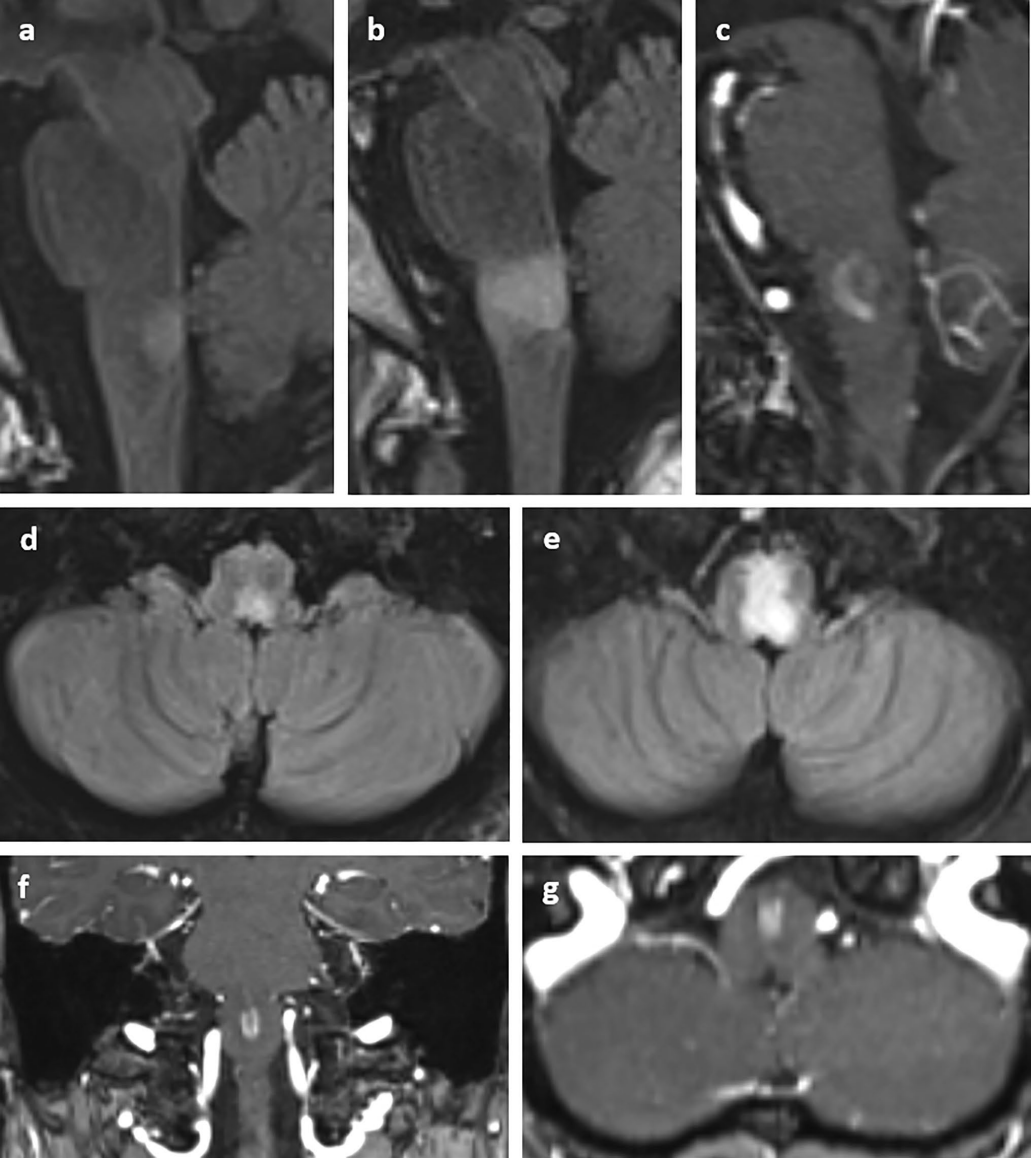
Cranial MRI 5 days after the onset of neurological symptoms revealed a T2/FLAIR-hyperintense lesion without gadolinium enhancement (not shown) in the dorsomedial medulla, localized in the nucleus tractus solitarii (a) Follow-up MRI 25 days later at the time of neurological deterioration showed enlargement of the same lesion (b), now with gadolinium enhancement (c). d, e Corresponding T2/FLAIR-weighted axial sections at day 5 after symptom onset and 25 days later. Panels f, g Gadolinium enhancement of the lesion at follow-up MRI in coronal (f) and axial (g) sections
